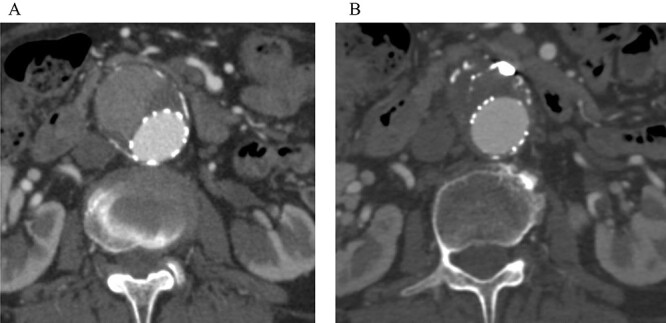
Post-embolization CT demonstrated that the sac shrank with no evidence of a continuing endoleak. (A) Pre-embolization CT showed a contrast effect in the sac. (B) Post-embolization CT showed no contrast effect in the sac.
